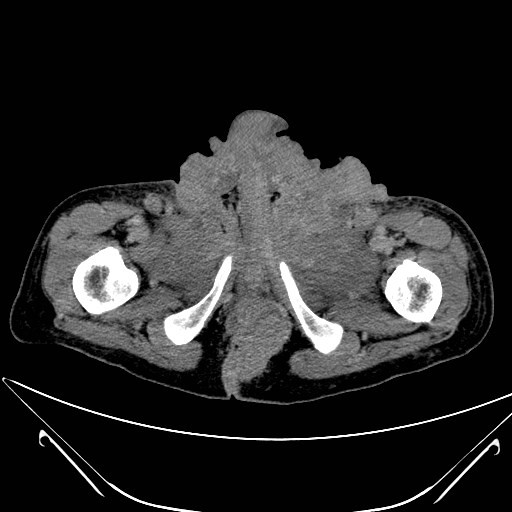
Extract of pelvis computed tomography scan showing a destructive, polycyclic growing tumor. This is a picture of a computed tomography scan that we made to exclude bone metastasis. It shows the tumor mass, growing from the anal region (below in the picture) to the groin region (above). Furthermore it shows the local destructive grow-pattern.
